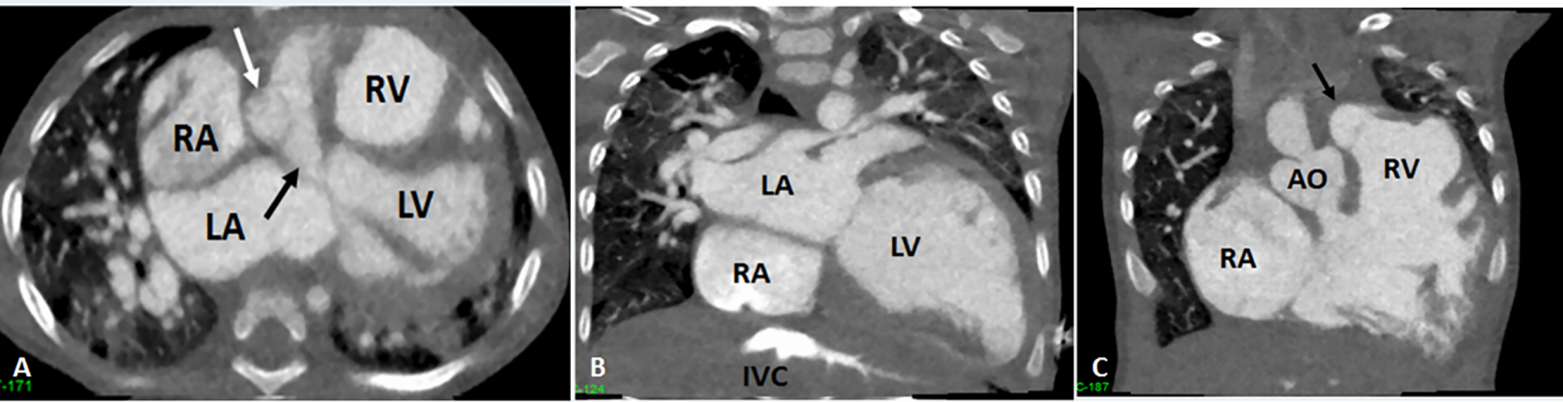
Cardiac computed tomography (CT) angiography showing double outlet right ventricle and ventricular septal defect (VSD). A: 2D axial CT image showing the four-chamber view with sub-aortic VSD (black arrow). B: 2D coronal CT image showing left atrium (LA), right atrium (RA) and left ventricle (LV). C: 2D coronal CT image showing that aorta (AO) and pulmonary artery (black arrows) all arise from right ventricle (RV). IVC-inferior vena cava, white arrow in A refers to ascending aorta.
